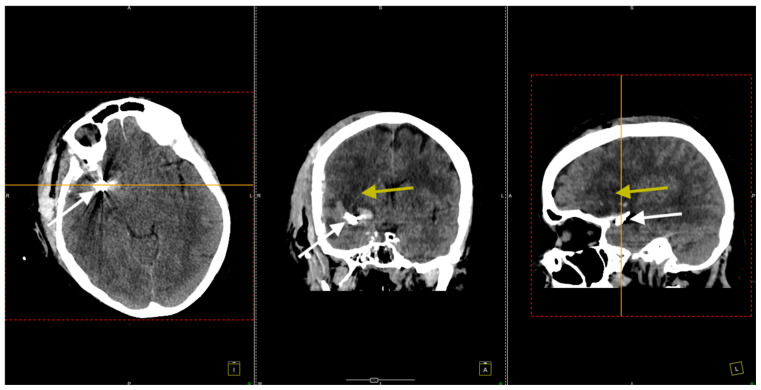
Postoperative CT scan. All three sections, transversal (left image), coronal (middle image), and sagittal (right image), show clip placement (white arrows) and sequelae from the ischemic stroke. The yellow arrows indicate areas of hypodensity corresponding to ischemic stroke sequelae in the right frontotemporal region.
